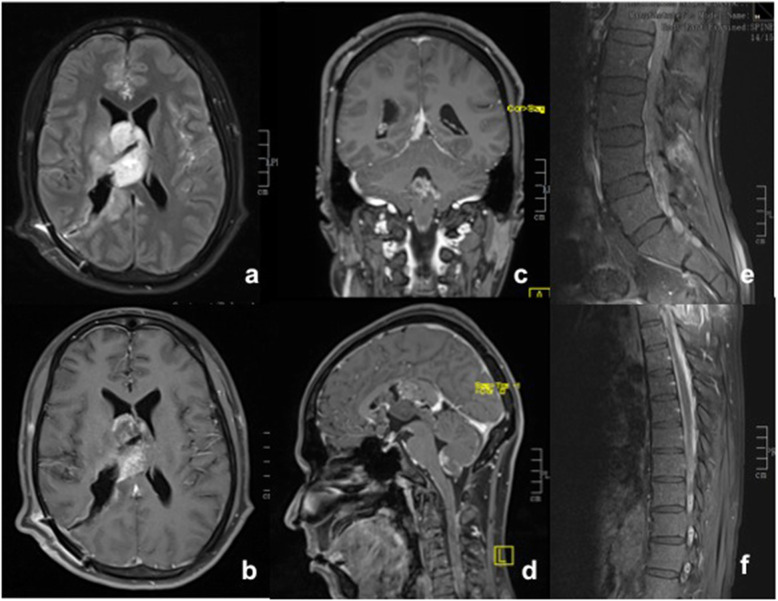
MRI of brain showed the residual right ventricular tumor mass infiltrating into right thalamus, posterior limb of right internal capsule, and reaching across the midline into the medial part of the left thalamus, causing mass effect over the third ventricle and mild upstream dilatation of the lateral ventricles. Abnormal enhancement was seen along the interpenduncular cistern, prepontine, perimesencephalic basal cistern, Sylvian fissures, cortical sulci, floor of fourth ventricle, and tentorium cerebelli, suggesting leptomeningeal spread. a T2 FLAIR axial section. b–d T1 contrast axial, coronal, and sagittal images. e, f Diffuse leptomeningeal enhancement along pial surface of spinal cord and along the nerve roots of cauda equina without any focal intramedullary lesion
